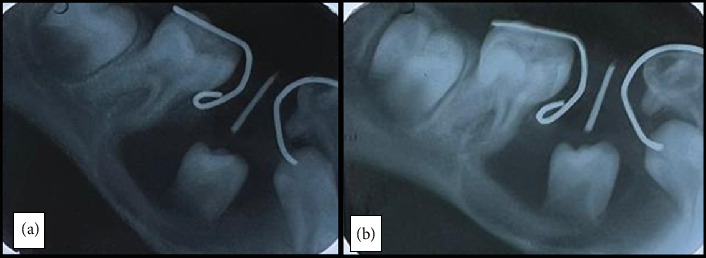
Postoperative radiographs at (a) 4 and (b) 8 weeks, respectively.
